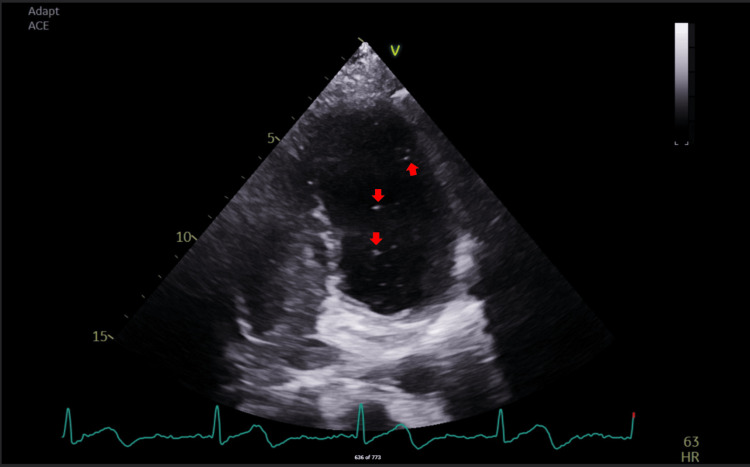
Transthoracic echocardiography (apical four-chamber view) shows microbubbles within the left ventricle following the administration of agitated saline contrast (bubble study; red arrows).
